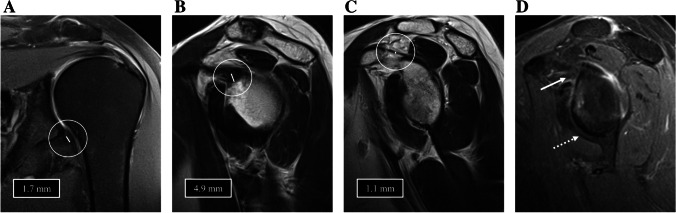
Images of the left shoulder of a 45-year-old female without clinical and MR morphological signs of ACS. A Coronal PD-weighted image showing a regular capsule at the axillary recess without thickening (1.7 mm) or hyperintensity. B Sagittal T2-weighted image shows a slightly thickened capsule at the rotatory interval (4.9 mm) and C a regularly thin CHL (1.1 mm) as well as non-obliterated subcoracoid fat. D T1-weighted fat saturated contrast-enhanced sagittal image is negative for contrast enhancement in the axillary recess (dotted arrow) and rotatory interval (arrow)
